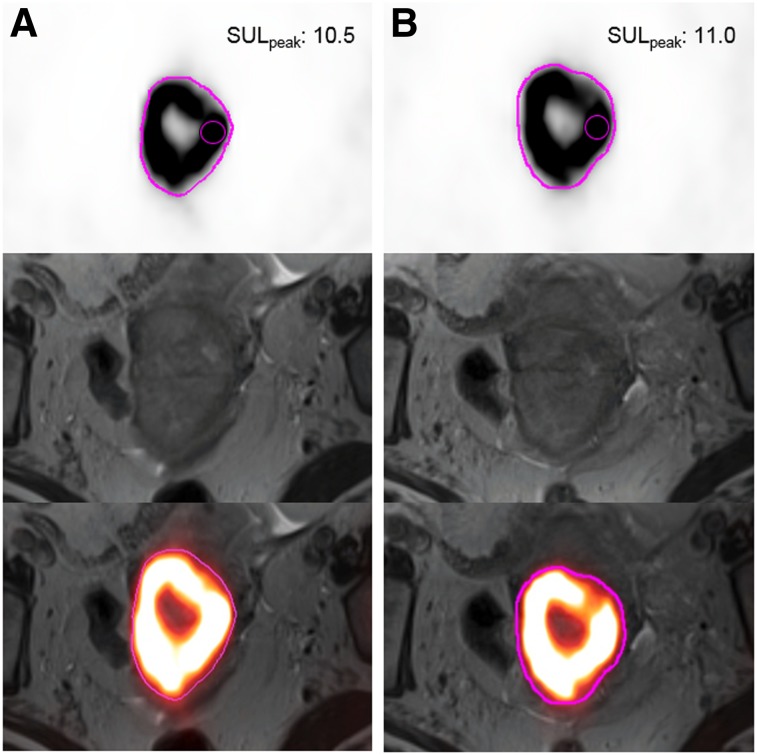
SUL_peak_ repeatability from PET image analysis. A 65-y-old woman with biopsy-proven squamous cell carcinoma of cervix was imaged with 24 h between session 1 (A) and session 2 (B). Transaxial PET images with manual whole-tumor contours (pink), T2-weighted MR images, and fused PET/MR images are shown from top to bottom for each imaging session. Note intersession contour similarities. SUL_peak_ (small pink sphere) was located in same tumor region for both sessions, with similar values.
